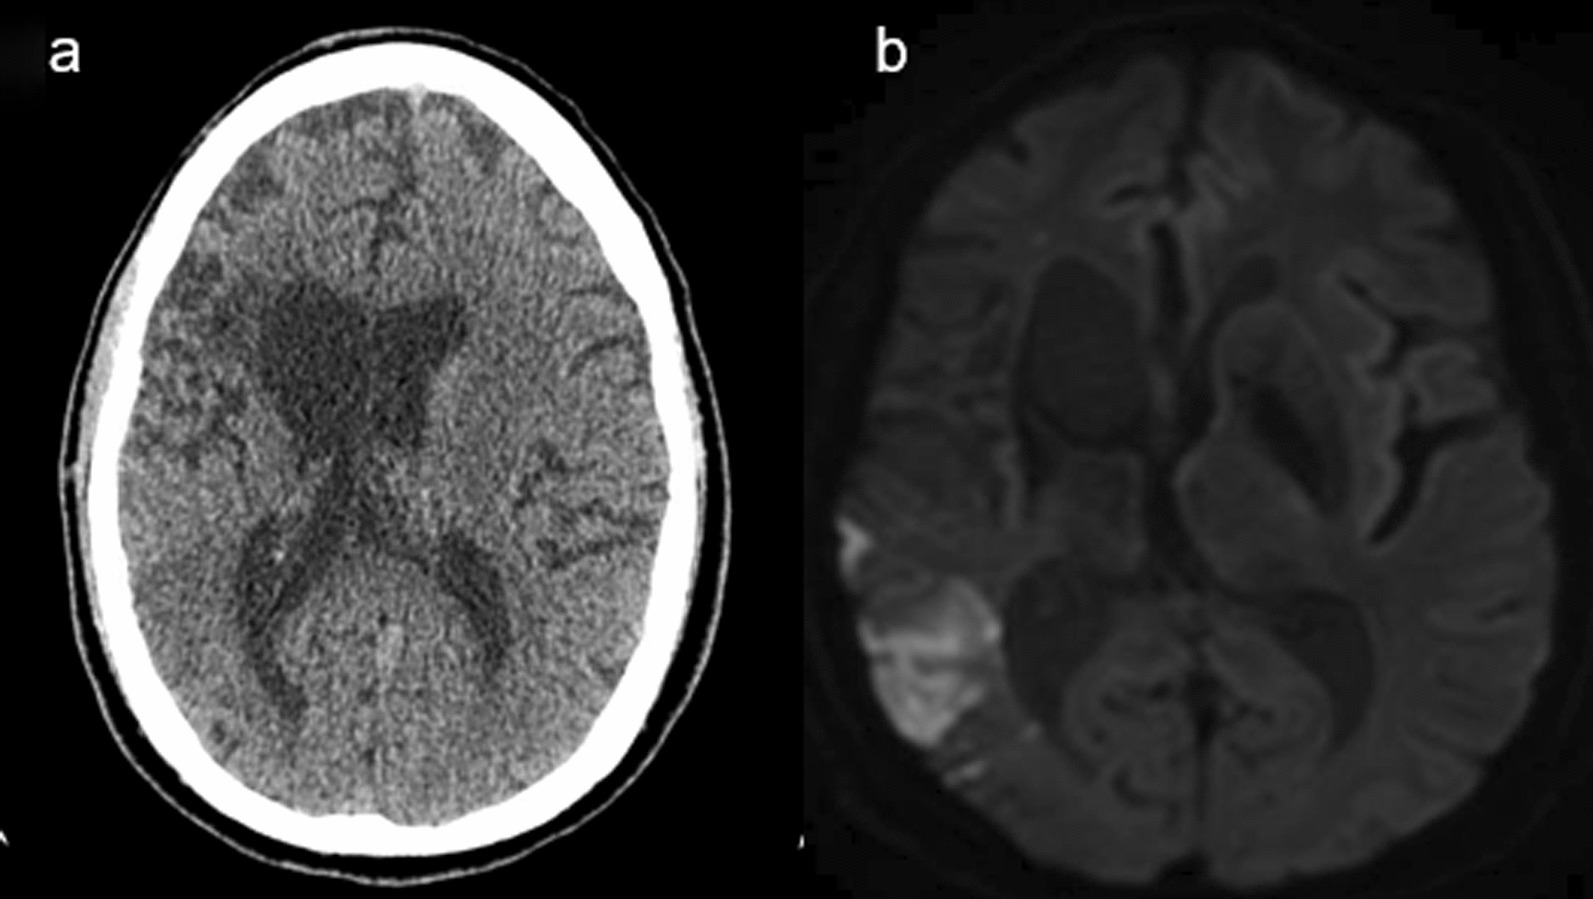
a Computerized tomography of the head without contrast showing chronic right middle cerebral artery (MCA) territory infarct including an area of evolving acute/subacute left temporal and parietal lobe ischemia. Shown better on b magnetic resonance imaging (MRI) showing acute right MCA territory infarct involving the right temporal lobe, right parietal lobe, and right precentral gyrus
